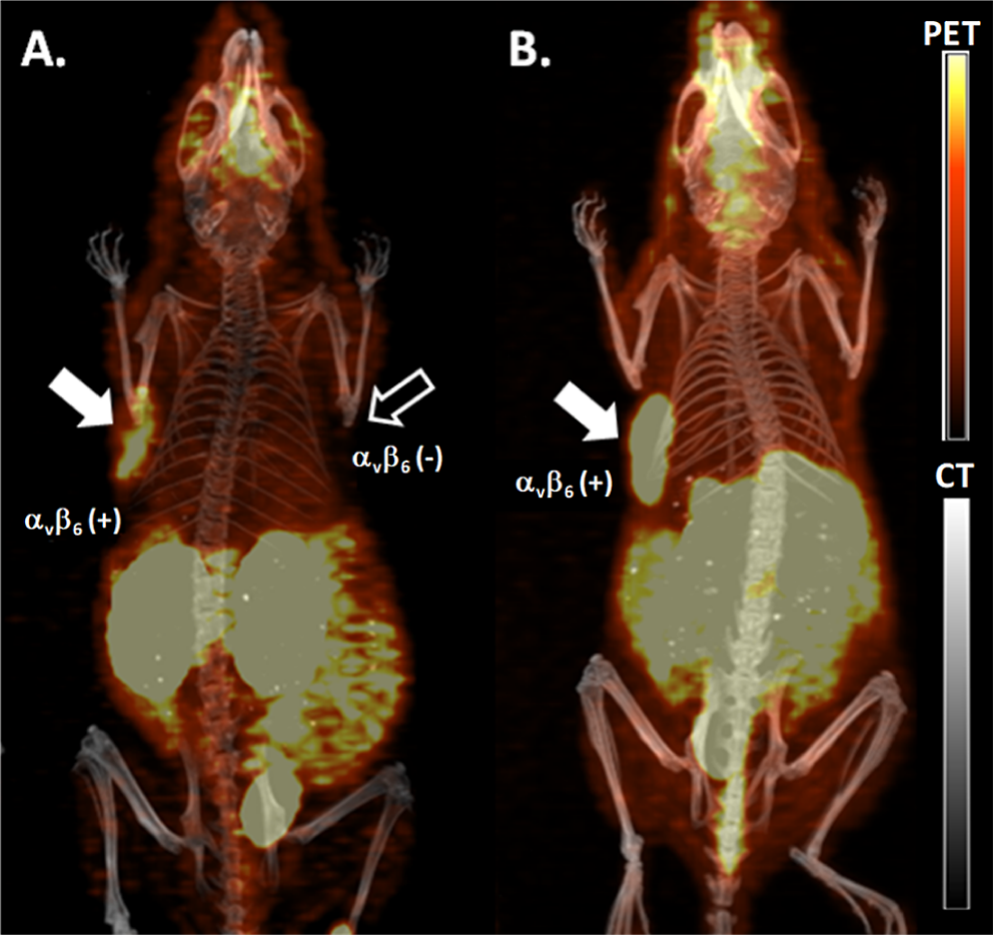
Maximum intensity projections (MIP) of PET/CT
